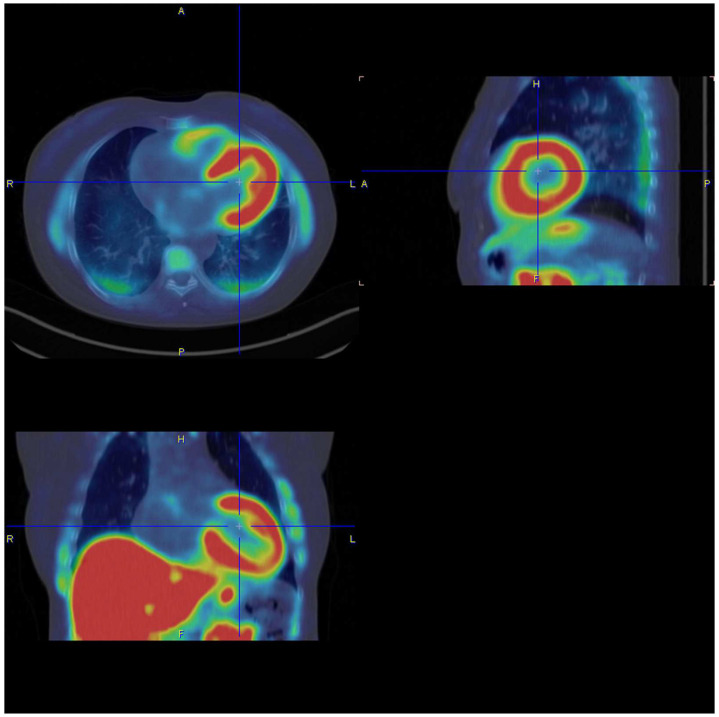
18F-florbetaben-PET/CT scan of a patient with cardiac AL amyloidosis (image by courtesy of [60]).
